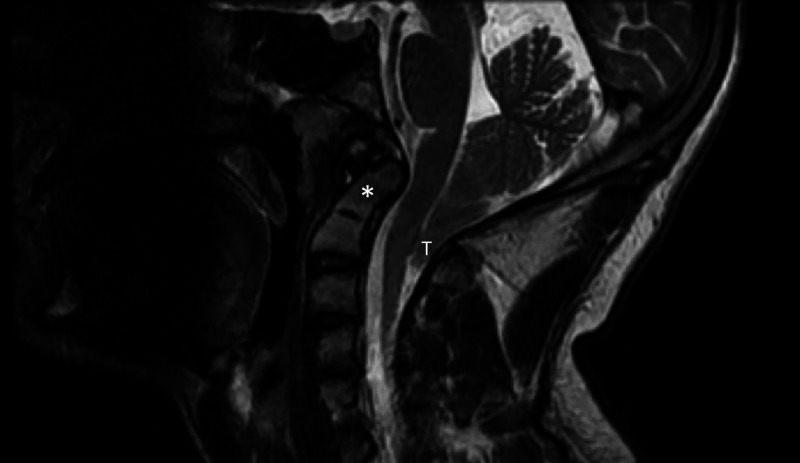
T2-weighted, sagittal MRI Note the herniated cerebellar tonsils (T) indicate a CM-1 and the retroverted odontoid process (asterisk). MRI: magnetic resonance imaging, CM-1: Chiari 1 malformation
